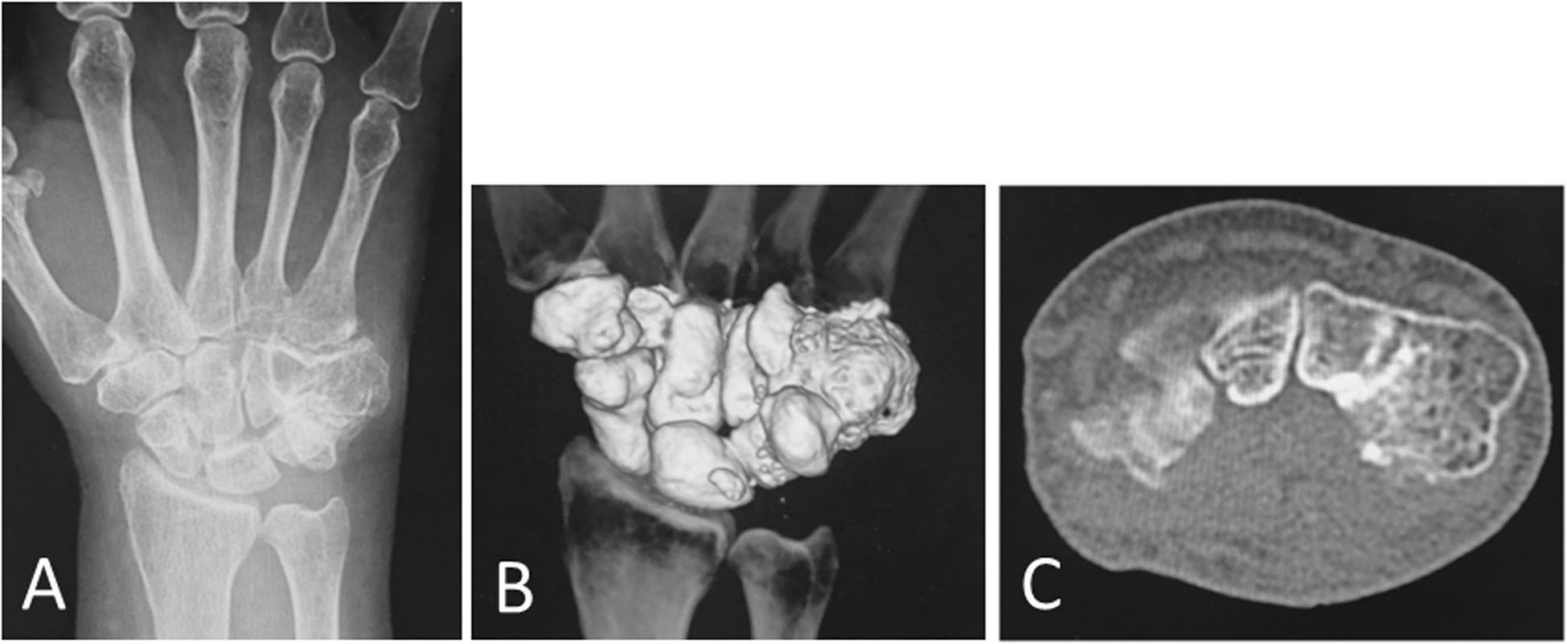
Plain radiograph (a) and computed tomography images (b, c) 6 months after surgery
